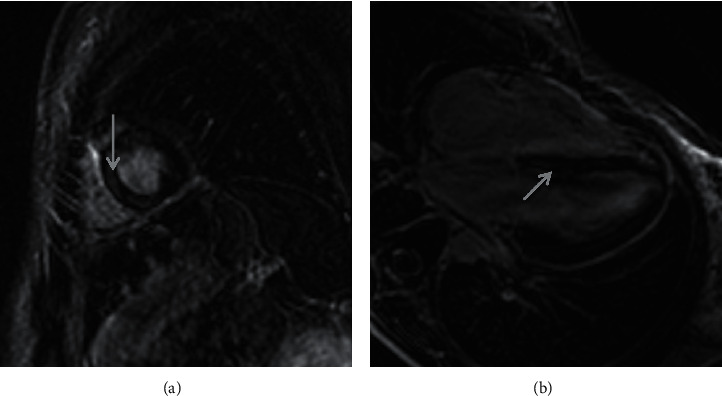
Initial T1-weighted delayed gadolinium-enhanced magnetic resonance images in (a) short axis and (b) four-chamber views demonstrating patchy midmyocardial delayed contrast enhancement in the anterior, inferior, and septal walls (arrows).
